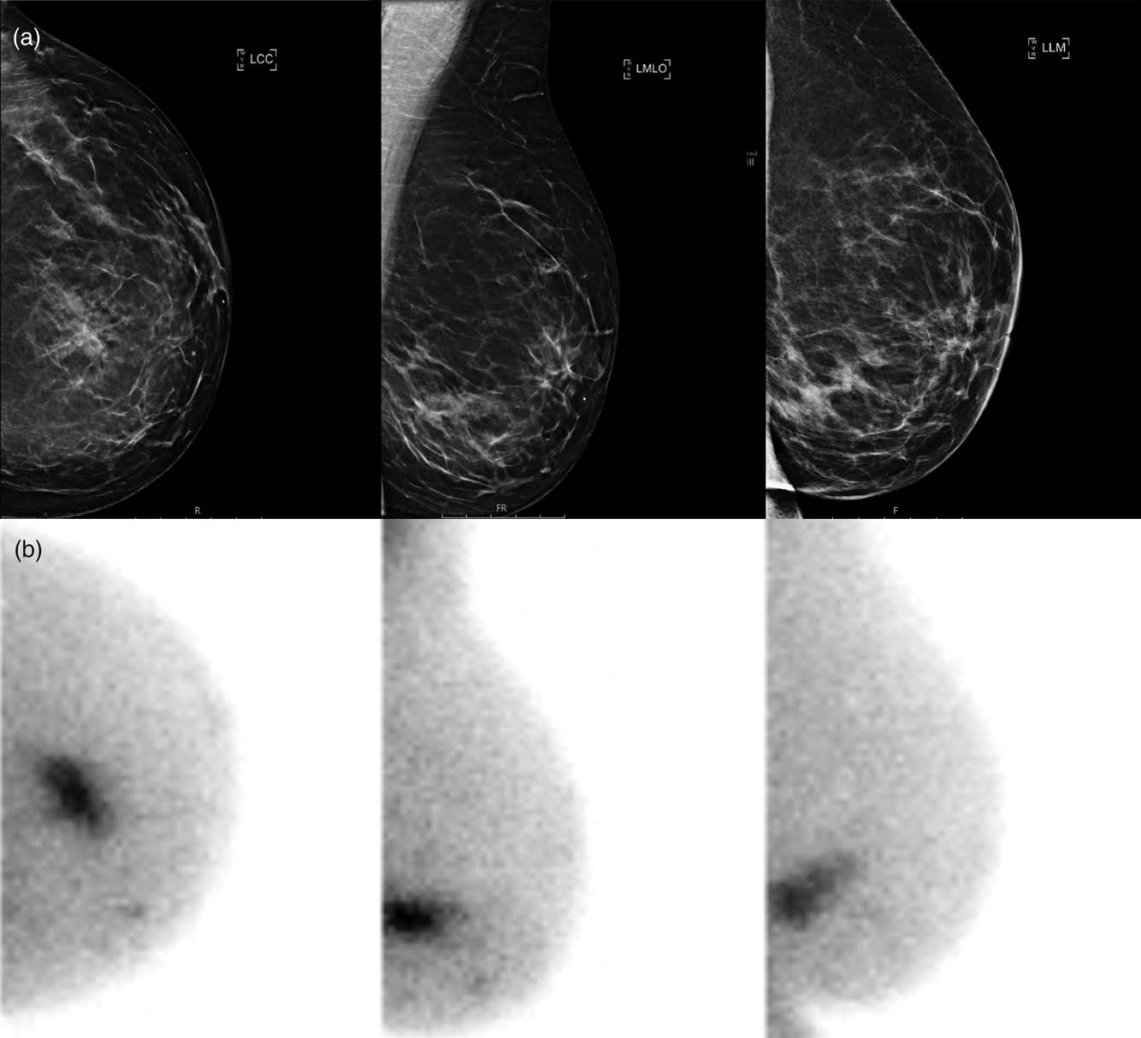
Breast imaging of a 56-year-old patient with left-sided breast cancer, detected at 1-year follow-up after treatment for right-sided breast cancer. With digital mammography, more glandular tissue was described in the medial lower quadrant (BI-RADS 3) (a), but no mass could be distinguished and ultrasound was negative. Molecular breast imaging (MBI; Dilon 6800 gamma camera) revealed suspicious sestamibi uptake in the medial lower quadrant over an area of 36 mm (b). MBI-guided biopsy was conducted, showing invasive carcinoma grade 2 with a lobular growth pattern, hormone receptor-positive, HER2-negative.
